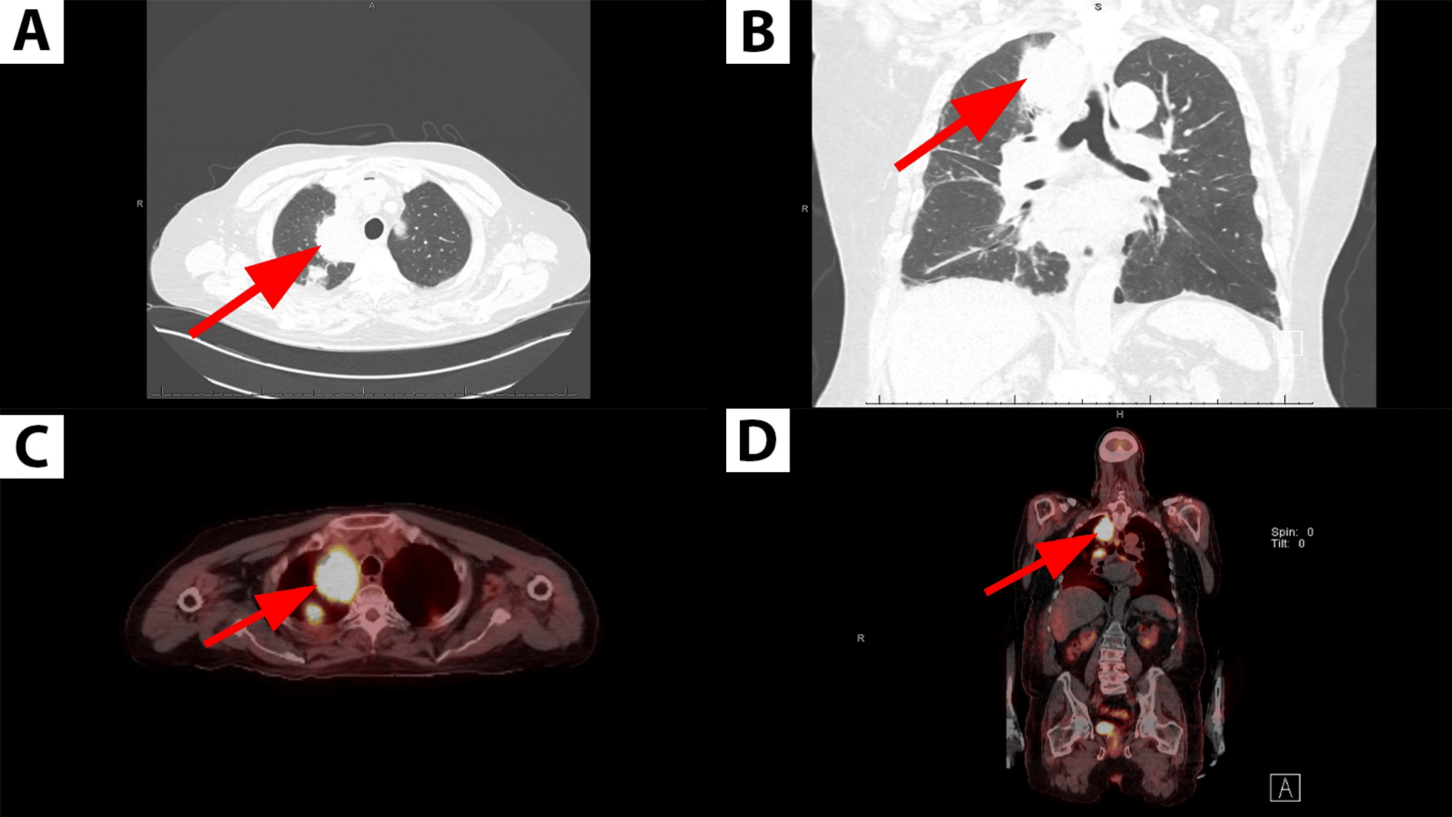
Initial axial and coronal images of chest CT and PET-CT. A. Axial view of chest CT showing a right apical lung mass (red arrow) accompanied by multiple bilateral solid nodules. B. Coronal view of chest CT showing the irregular and heterogeneous right apical mass. C. Axial view of the PET-CT demonstrating hypermetabolic activity of the right apical lung mass (red arrow). D. Coronal view of the PET-CT highlighting hypermetabolic mass and associated nodule.
